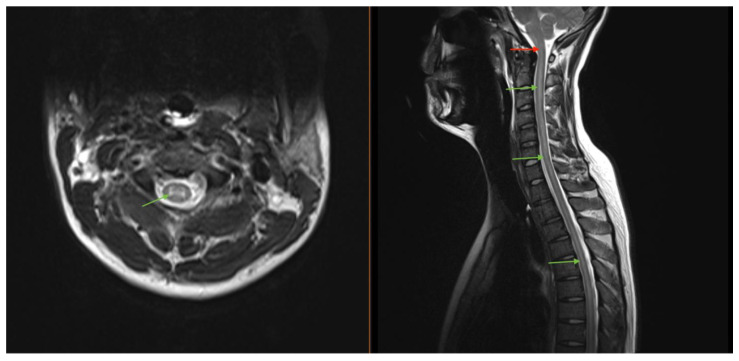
MRI imaging of our patient who was found to have a longitudinally extensive gray matter lesion (green arrows) extending cranio-caudally from the brainstem through the lumbar spinal cord. The lesion involvement of the dorsal medulla (red arrow) was thought to represent the pathologic etiology of the DBN seen in our case.
